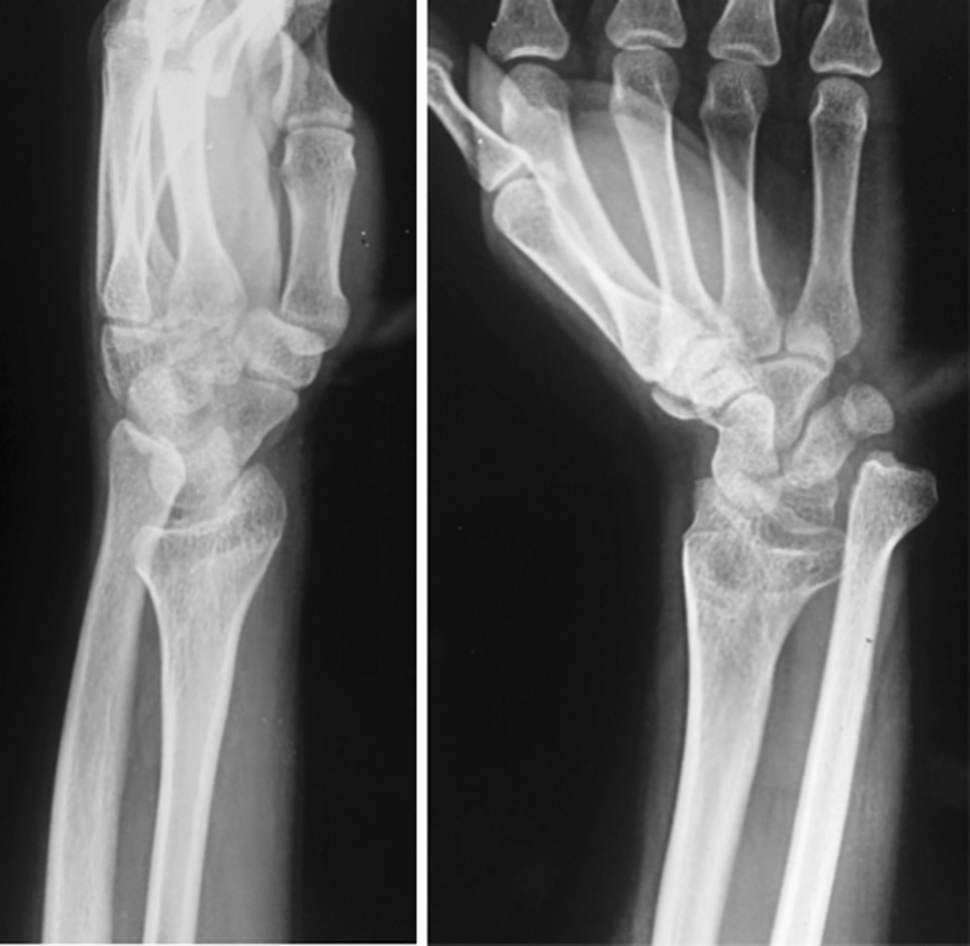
Preoperative radiographs of a 21-year-old male with neglected epiphyseal injury of the distal end of the radius with DRUJ disruption and dorsal ulnar subluxation
